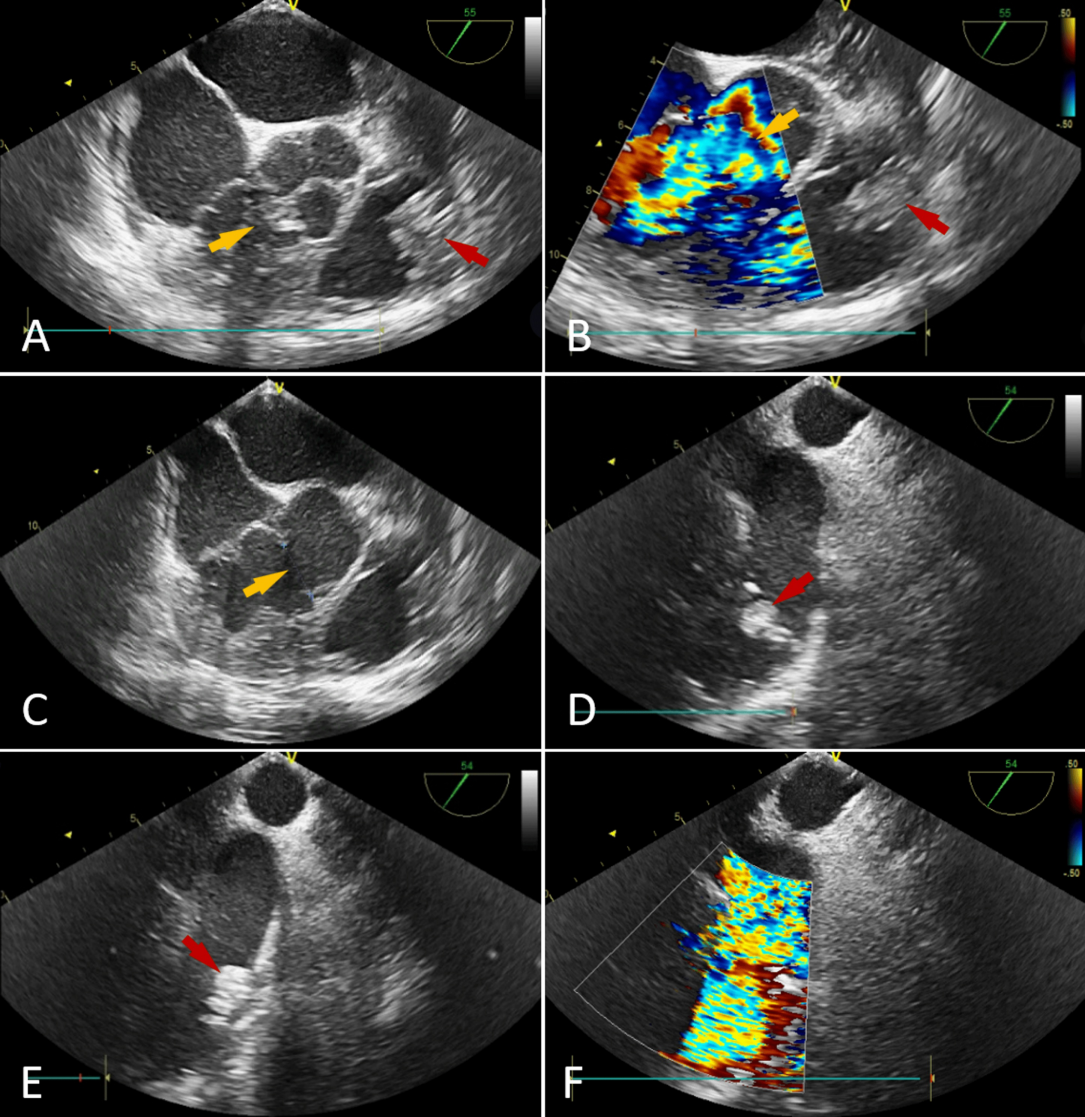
Transesophageal echocardiographic view. Mid-esophageal aortic valve short axis showing a perimembranous ventricular septal defect (yellow arrow) with a large vegetation on the pulmonary valve (red arrow) (A). Mid-esophageal aortic valve short axis showing a perimembranous ventricular septal defect (yellow arrow) with left-to-right shunting on color Doppler, along with a large vegetation on the pulmonary valve (red arrow) (B). Mid-esophageal aortic valve short axis showing a perimembranous ventricular septal defect measuring 19 mm (yellow arrow) (C). Upper-esophageal aortic arch short axis showing a large vegetation (red arrows) on the pulmonary valve (D and E). Upper-esophageal aortic arch short axis showing severe pulmonary regurgitation (F).
